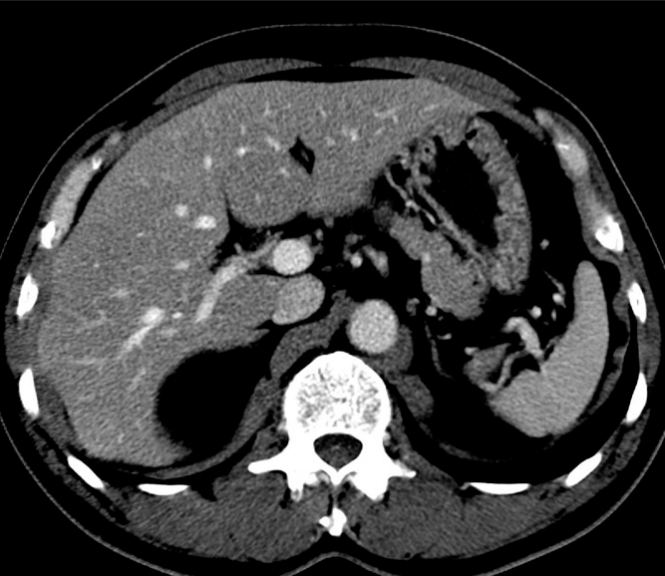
The CT image obtained 15 months later discloses no nodule.
